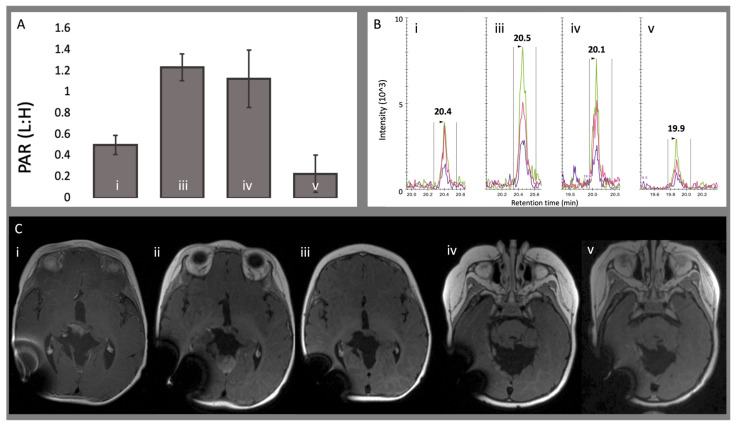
Longitudinal multimodal surveillance demonstrating changes in CSF endogenous light signal and correlated MRI findings in a treated ATRT patient (1715). (A–C) depict matched surveillance timepoints, with labels i, iii, iv, and v representing dates for which both CSF analysis and MRI were obtained (i = July 2021; iii = February 2022; iv = January 2023; v = April 2024). Subpanel ii corresponds to September 2021, a timepoint with MRI only and no CSF sample collected. (A) Peak area ratio (relative to heavy internal standard) demonstrating an increase at timepoint iii, followed by a progressive decline across subsequent surveillance timepoints. (B) Endogenous light signal chromatograms showing a parallel increase at timepoint iii with steady reduction thereafter. (C) Axial T1-weighted MRI with contrast. (Ci) demonstrates residual postoperative tumor. (Cii) shows significant interval tumor progression in the subvermian region, prompting escalation of chemotherapy followed by radiotherapy. (Ciii) demonstrates a marked reduction in tumor volume, with stability maintained on subsequent follow-up studies (Civ and Cv).
